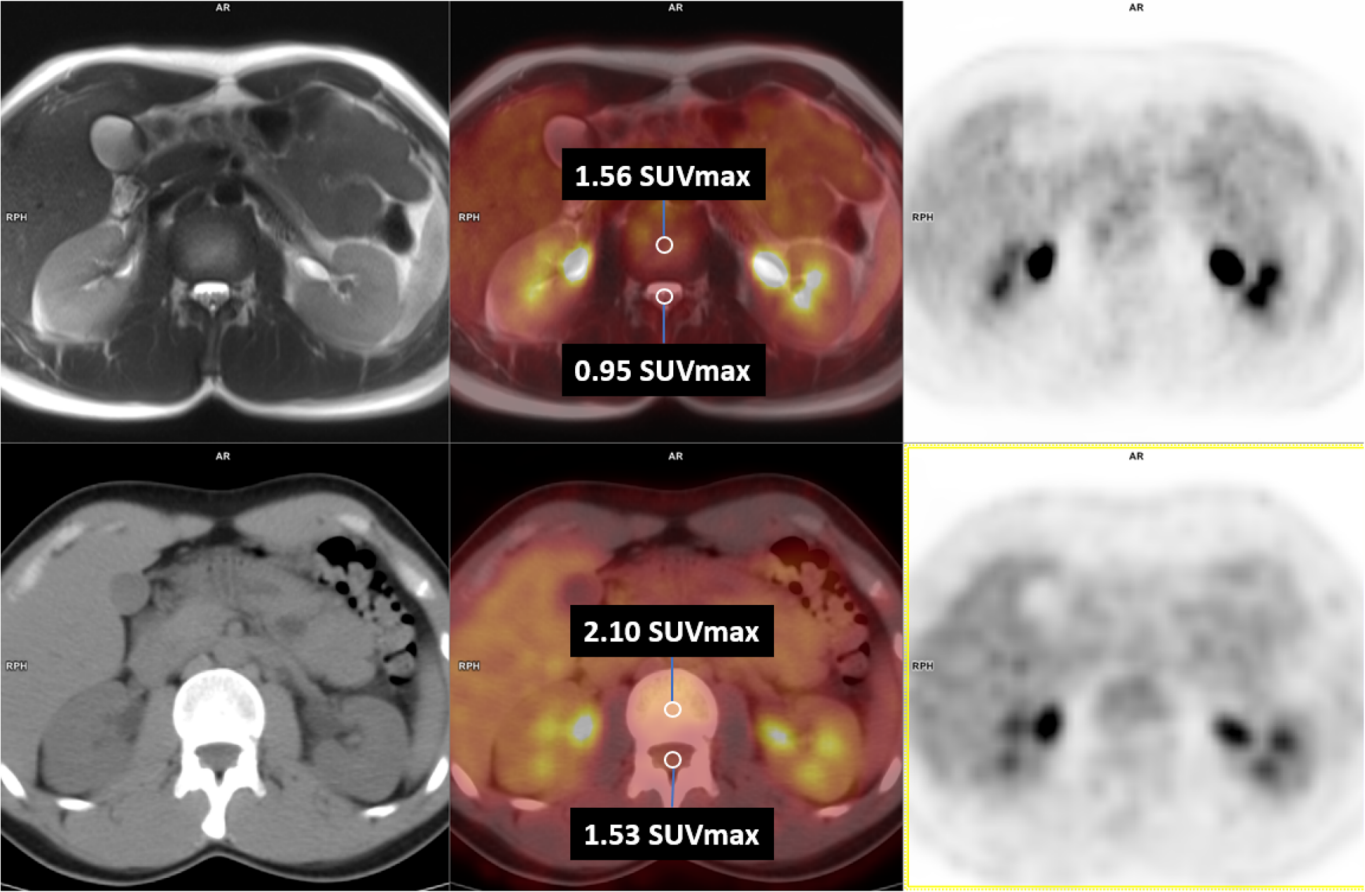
Example of a 3 mm radius ROI at T12 level. The ROI was centered on the spinal cord and the corresponding vertebral body bone marrow. Top row from left to right (MRI, PET/MRI, PET); bottom row from left to right (CT, PET/CT, PET)
